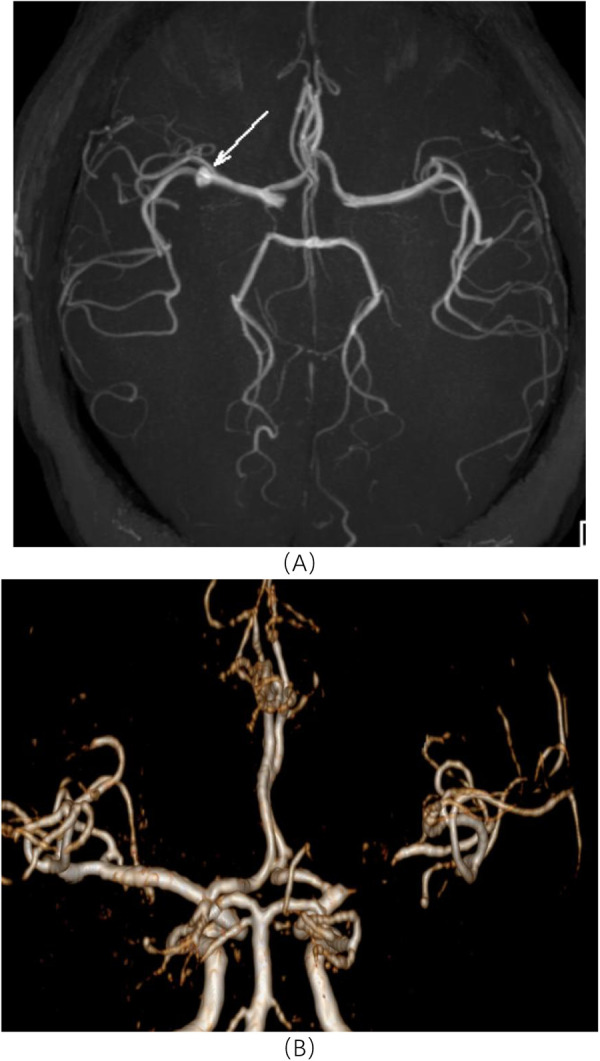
Radiographic image of a patient treated using endovascular embolization (Male patient, 67 years old). (A) represents preoperative head DSA images, and (B) represents postoperative 3D head DSA images.
